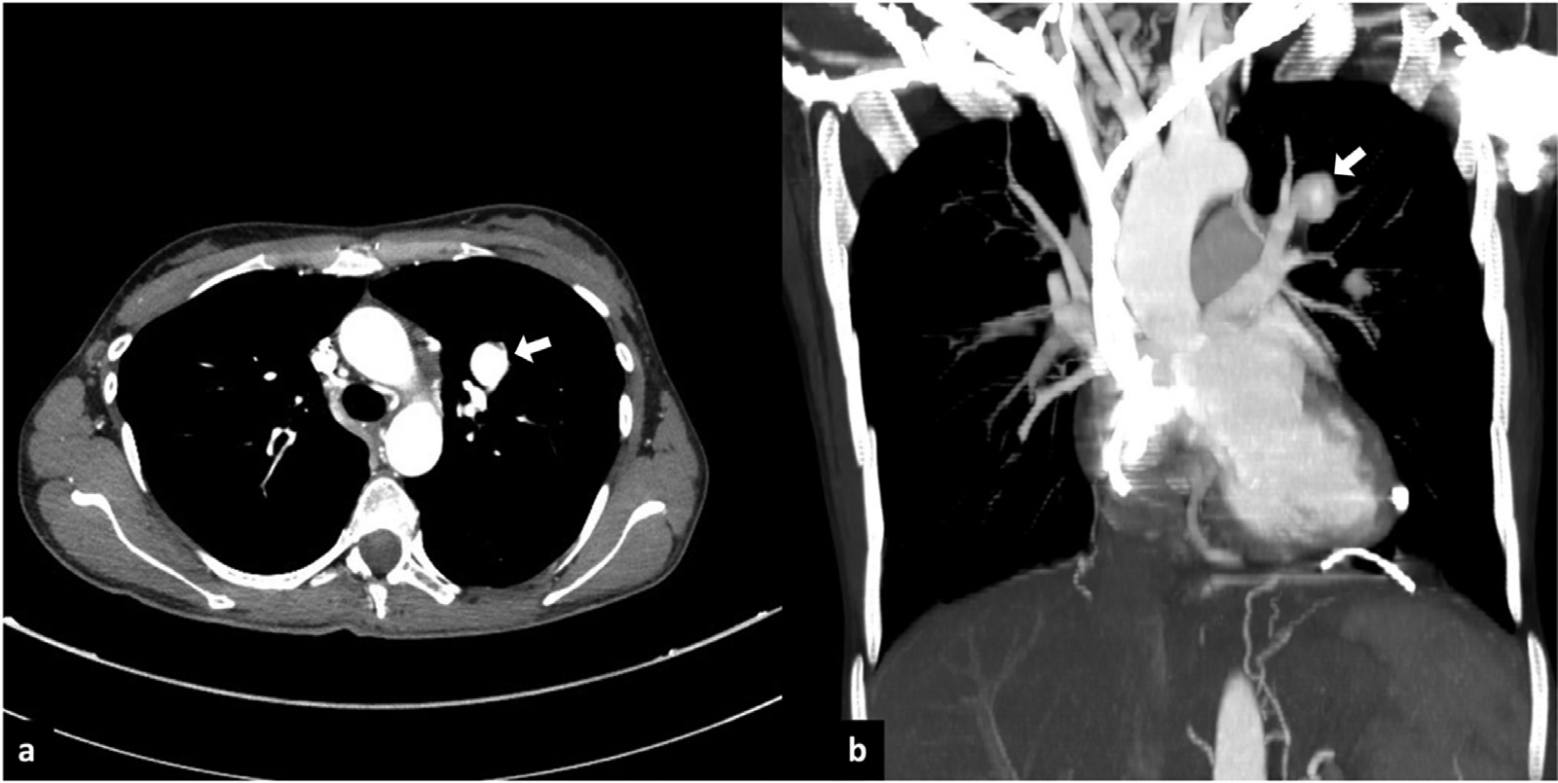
Computed tomography angiography (CTA) images. (A) Axial and (B) coronal maximum intensity projection (MIP) reconstruction views show the presence of an aneurysmal sac originating from a segmental branch of the left pulmonary artery (white arrows).
